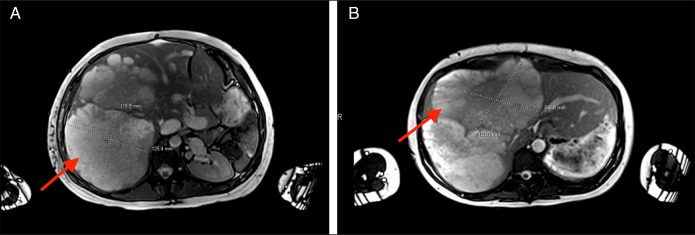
(A) Eight years after initial diagnosis and resection, magnetic resonance imaging (transverse cross-sectional view) of hepatic metastases of pancreatic SPEN (red arrow). (B) Eight years after initial diagnosis and resection, magnetic resonance imaging (transverse cross-sectional view) of hepatic metastases of pancreatic SPEN (red arrow).
